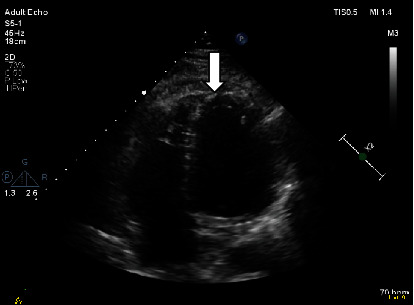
Apical 4 chamber views show pericardial thickening around the apex of left and right ventricles. White arrows; in addition to small pericardial effusion.
